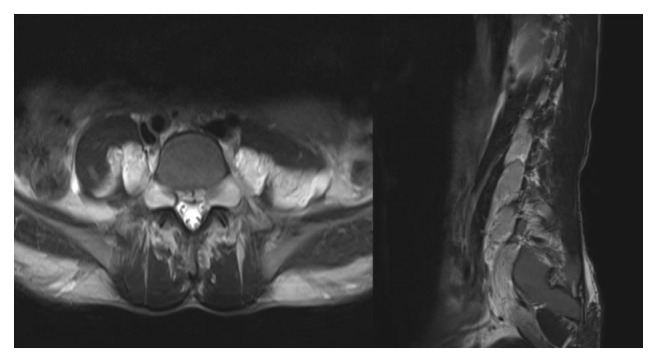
T2W MRI scan of the lumbar spine showing bilateral enlarged exit neural foramen along with extensive neurofibromas and bilateral plexiform neurofibromas.
